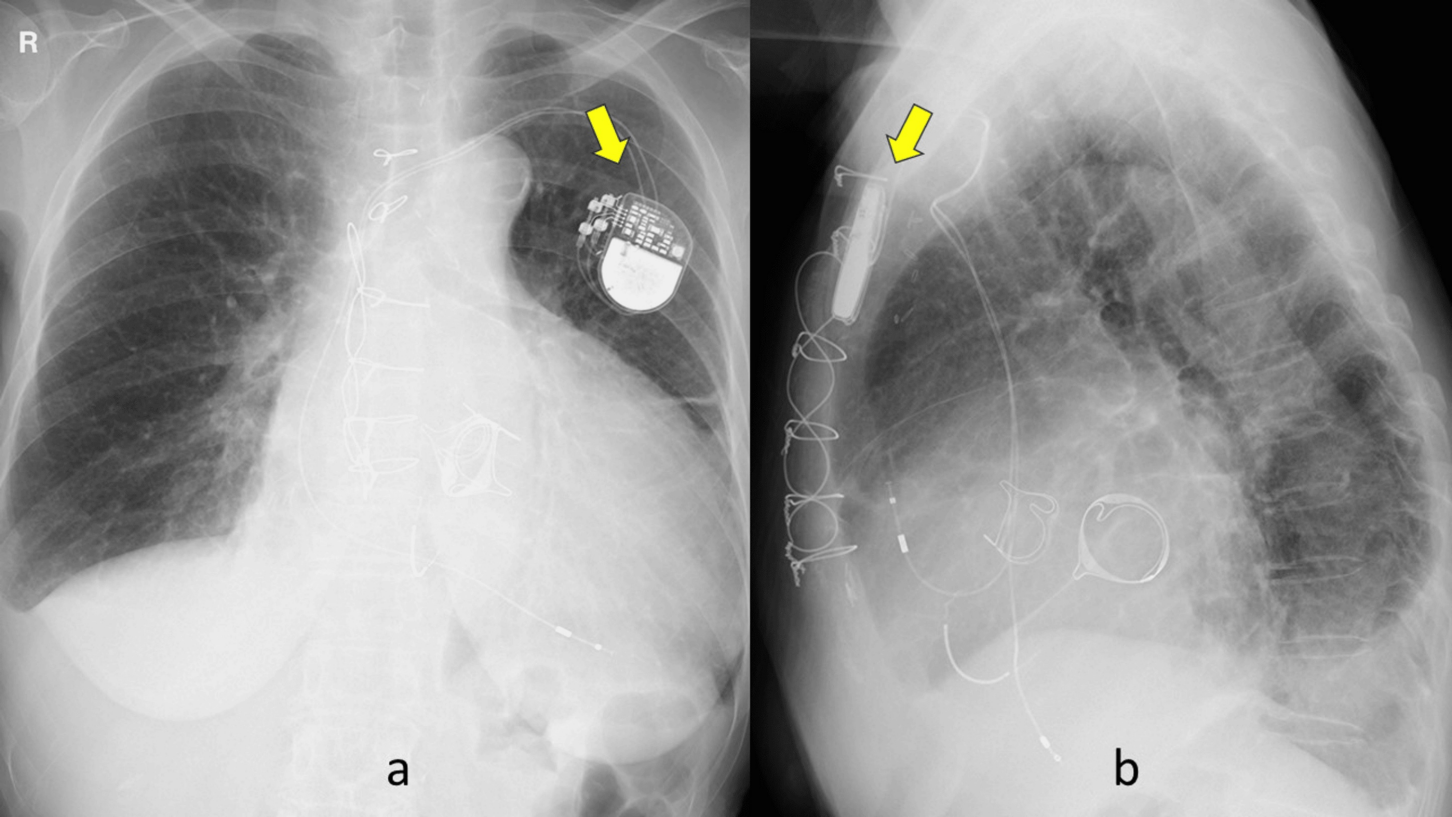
Chest X-ray films. Arrows show the implanted pacemaker. Ground glass opacity in the left upper lobe of the lung cannot be seen. (a) Frontal and (b) lateral.
